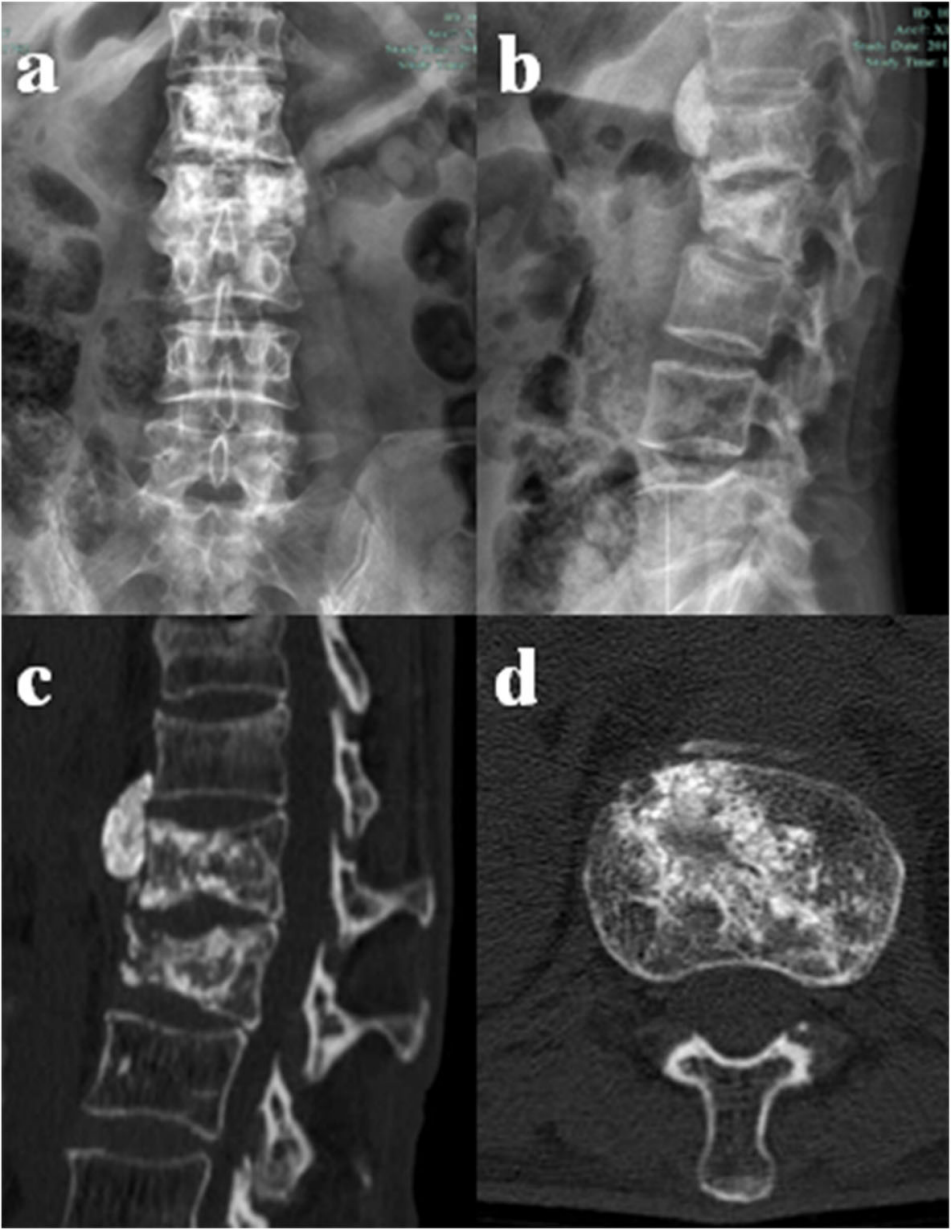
Radiographic data at 10 years after radiotherapy: a, b Radiograph showing sclerotic bone in the L1 and L2 vertebral body. The L2 vertebral body was compressed, which induced local kyphosis of the thoracolumbar spine. c, d CT scan demonstrating calcification or sclerotic bone without obvious osteolytic lesion in the L1 and L2 vertebral bodies
